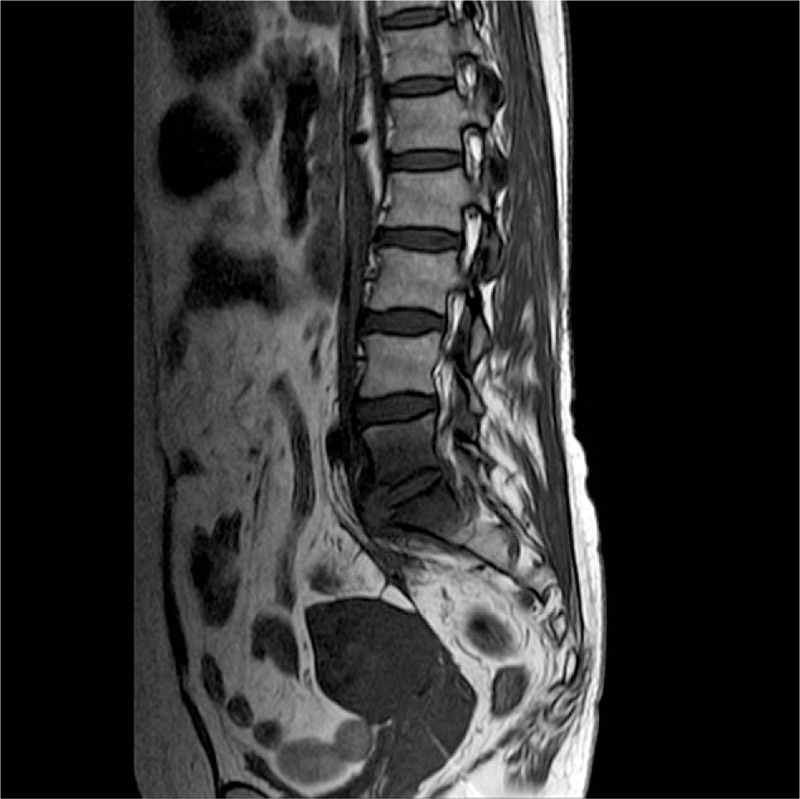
MRI: Lumbar pyogenic spondylodiscitis, enhancement of soft tissues surrounding the L5-S1 vertebrae (arrow). MRI = magnetic resonance imaging.
